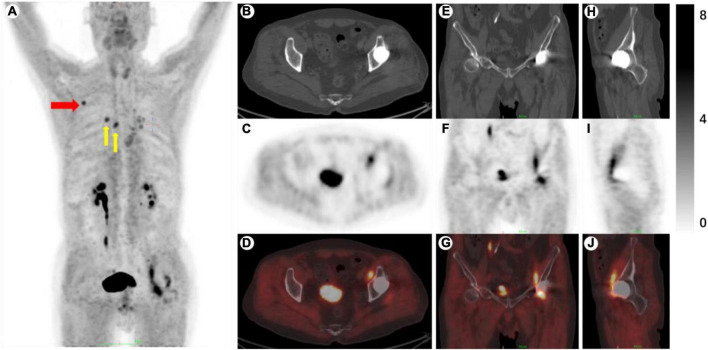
(A) MIP. (B–D) Axial images of CT, PET, and fused. (E–G) Coronal images. (H–J) Sagittal images. Red arrow, right scapular spine, SUVmax: 4.9. Yellow arrow, multiple calcified lymph nodes, SUVmax: 4.5.
